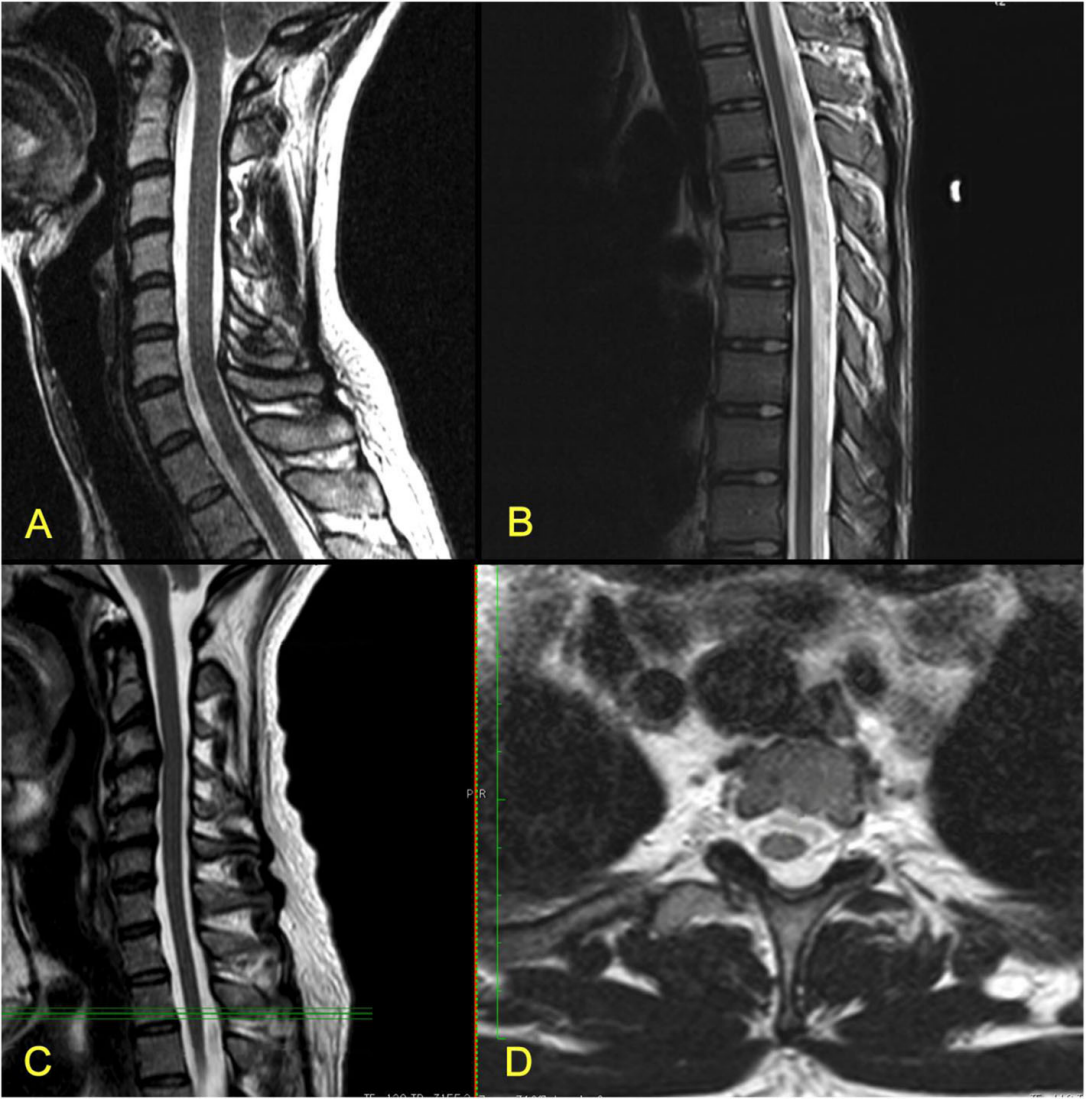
MRI cuts showing features of Tense Spinal Cord (A, C - cervical spine; B - thoracic spine) and Lateralized Spinal Cord (D, at the level marked with green lines in C)
